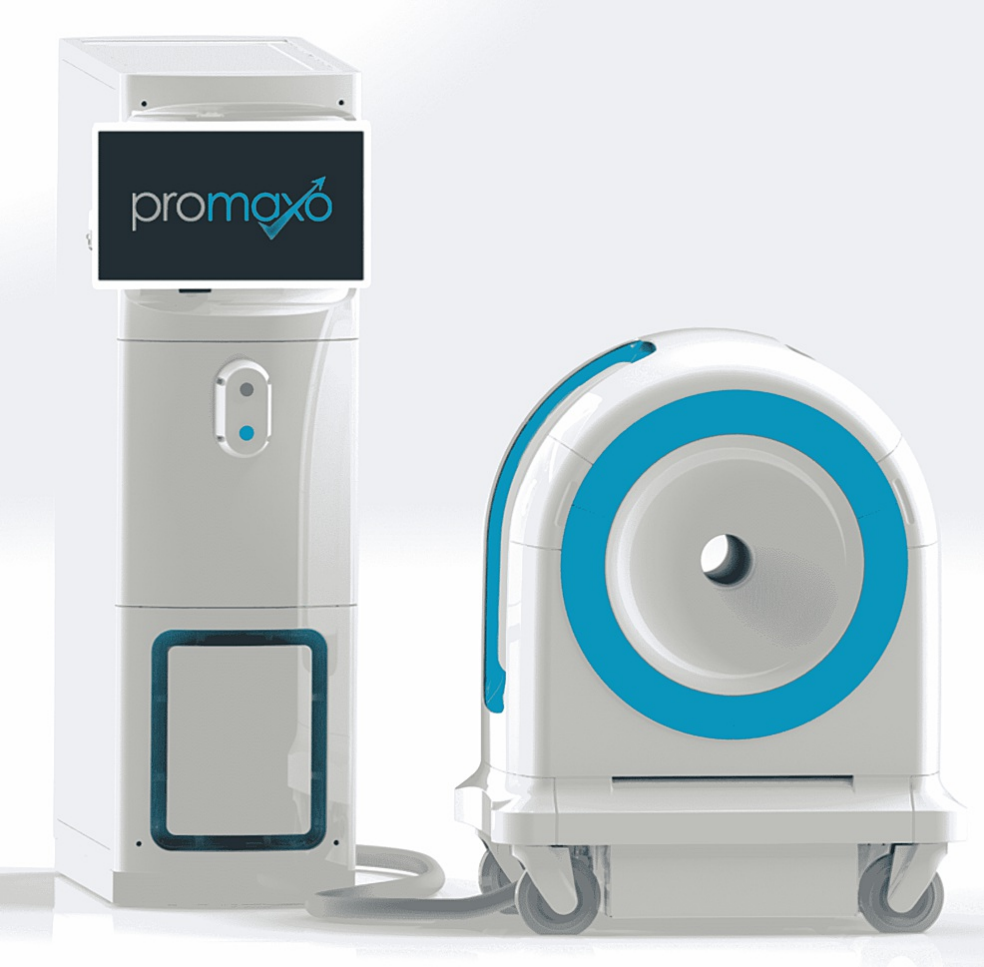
The Promaxo MRI system consisting of the magnet (ranging from 58mT to 74mT) and accompanying electronic rack with an attached graphical user interface (GUI). Promaxo Inc., Oakland, California, United States
